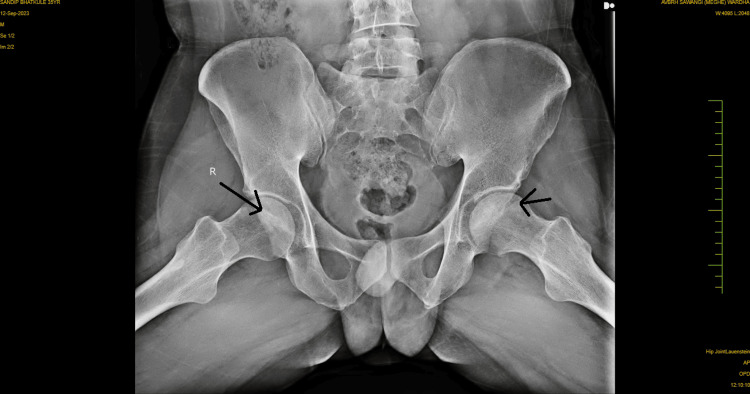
Lateral radiograph of both hips showing cysts on both hips
